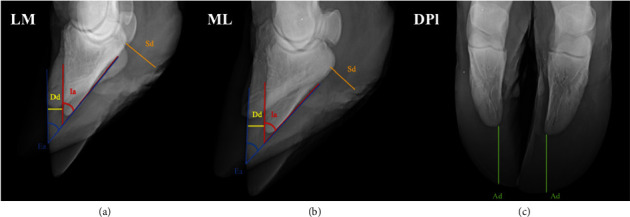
Radiographs of the third phalanx of the right pelvic limb of a Nelore bull in lateromedial (a), mediolateral (b), and plantarodorsal (c) exhibitions. Distance between the dorsodistal edge of the third phalanx and the dorsal wall of the hoof (Dd), distance between the solar edge of the flexor tubercle and the hoof (Sd), external angle over the silhouette of the dorsal edge of the hoof and the sole of the third phalanx (Ea), internal angle on the silhouette of the third phalanx (Ia), and distances between the apex of the hoof and the apex of the third phalanx in plantarodorsal (Ad).
